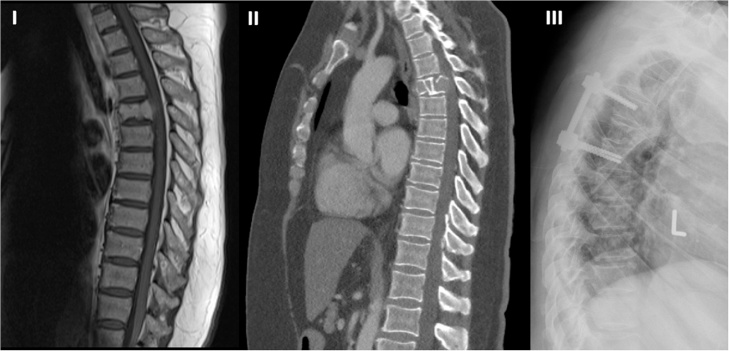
I) Preoperative sagittal T1 weighted magnetic resonance imaging showing a hypointense lesion with dorsal extrusion in T5 and less than 50% vertebral body collapse. II) Sagittal computed tomography scan showing expansile irregular osteolytic lesion of T5 vertebral body and involvement of the anterior and posterior wall. III) Lateral thoracic radiography after decompression of T5 and dorsal instrumented stabilization of T4–T6.
